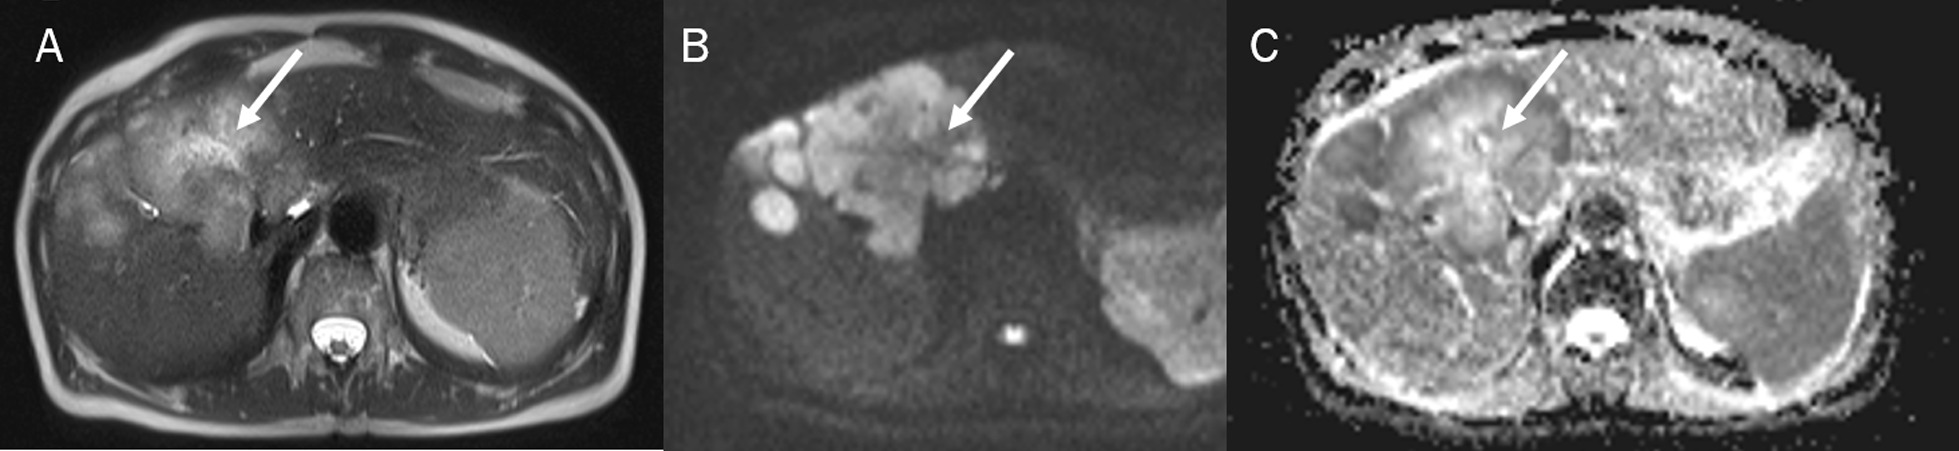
MRI assessment of liver colon rectal metastases. The lesions show (arrow) the TA appearance due to the central necrosis, with a central area of higher intensity compared to peripheral area of lesion on T2-W sequences (A) and DWI sequences (B, C)
